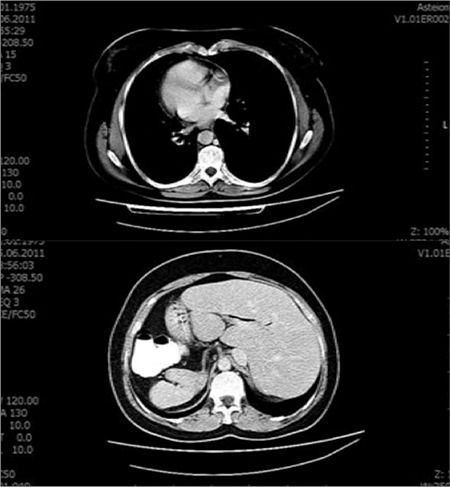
Computed tomography shows dextrocardia with situs solitus inversus (the heart on the right, the liver on the left side and spleen on the right side).
